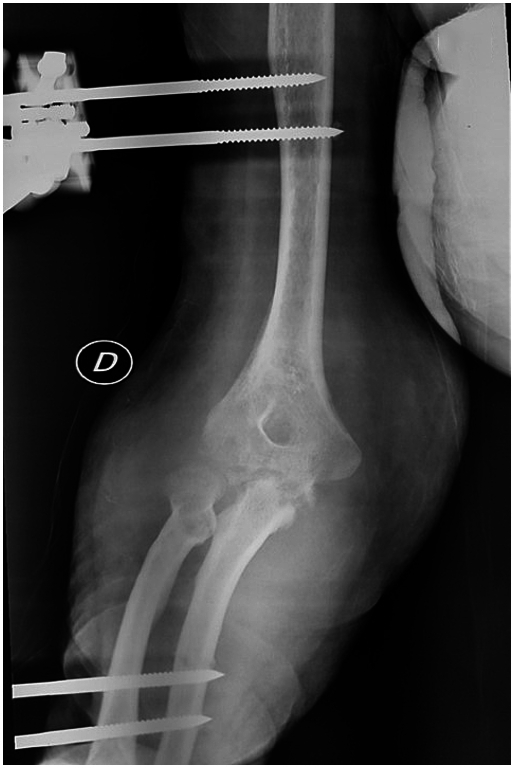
Anteroposterior radiograph of the right elbow demonstrating the utilization of an external fixator for stabilization.
